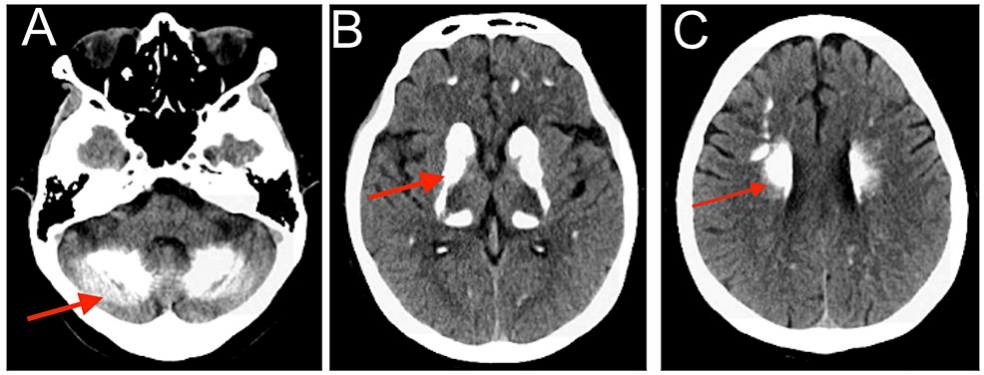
Head CT. Head CT without contrast demonstrating symmetric hyperdensities of the globus pallidi, caudate nuclei, dentate nuclei, and the periventricular and subcortical white matter (A-C, arrows). These findings along with the brain MRI were consistent with diffuse brain calcifications.
